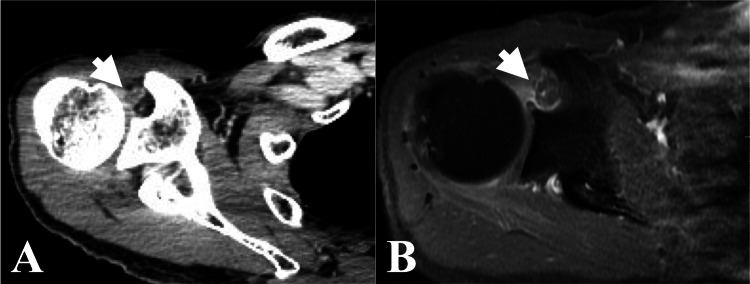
Case 3. Contrast-enhanced CT and MRI findings. (A) Axial CT of CK-SBRT planning showing a contrast-enhanced lesion at the rotator interval of the right shoulder joint. (B) Just before CK-SBRT, axial MRI showing a contrast-enhanced lesion at the rotator interval of the right shoulder joint. CT: computed tomography; MRI: magnetic resonance imaging; CK-SBRT: stereotactic body radiotherapy using CyberKnife
